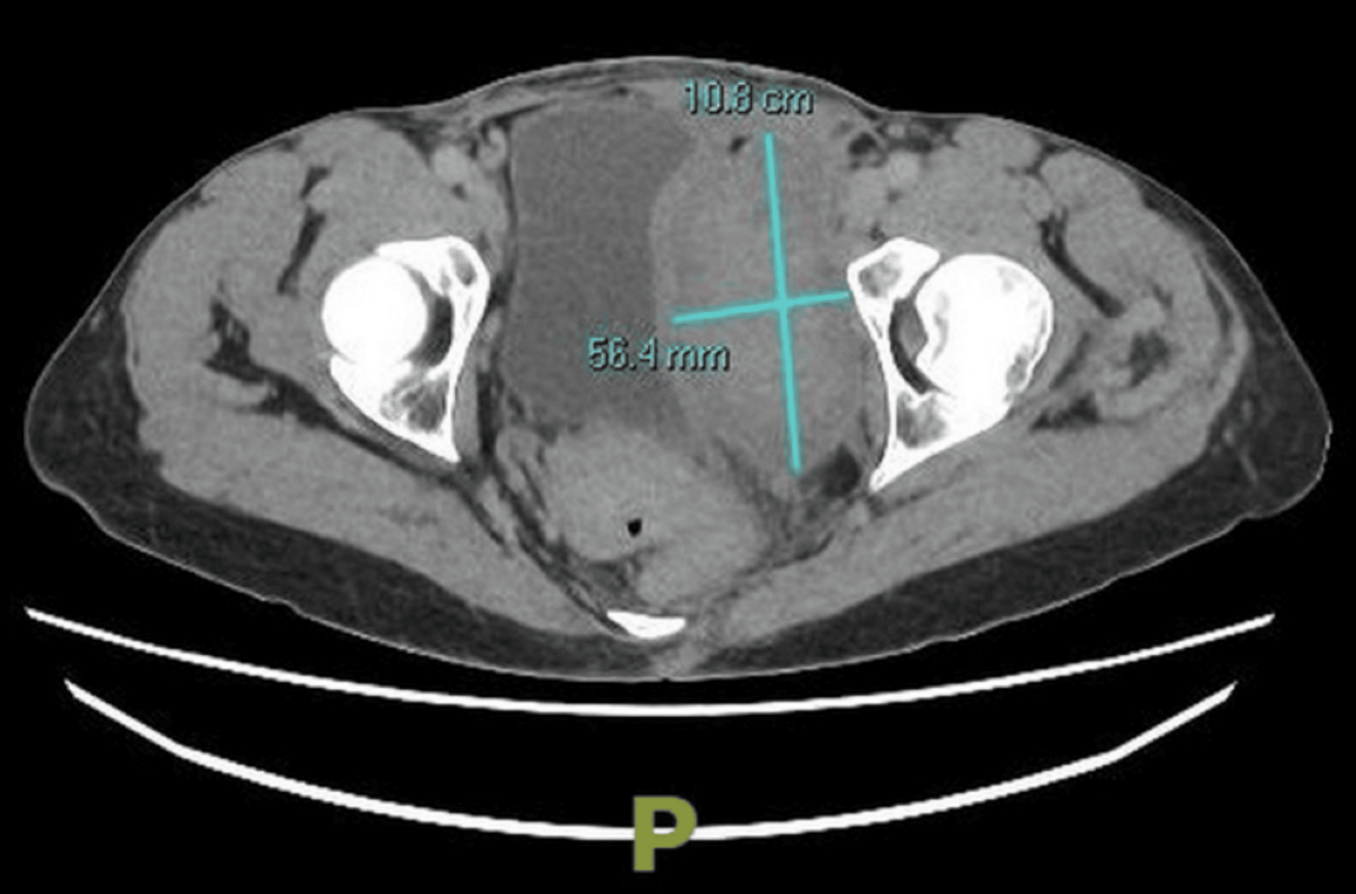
Axial spiral CT scan with arterial and portovenous contrast phases showing reduction in attenuation indicating resolution
